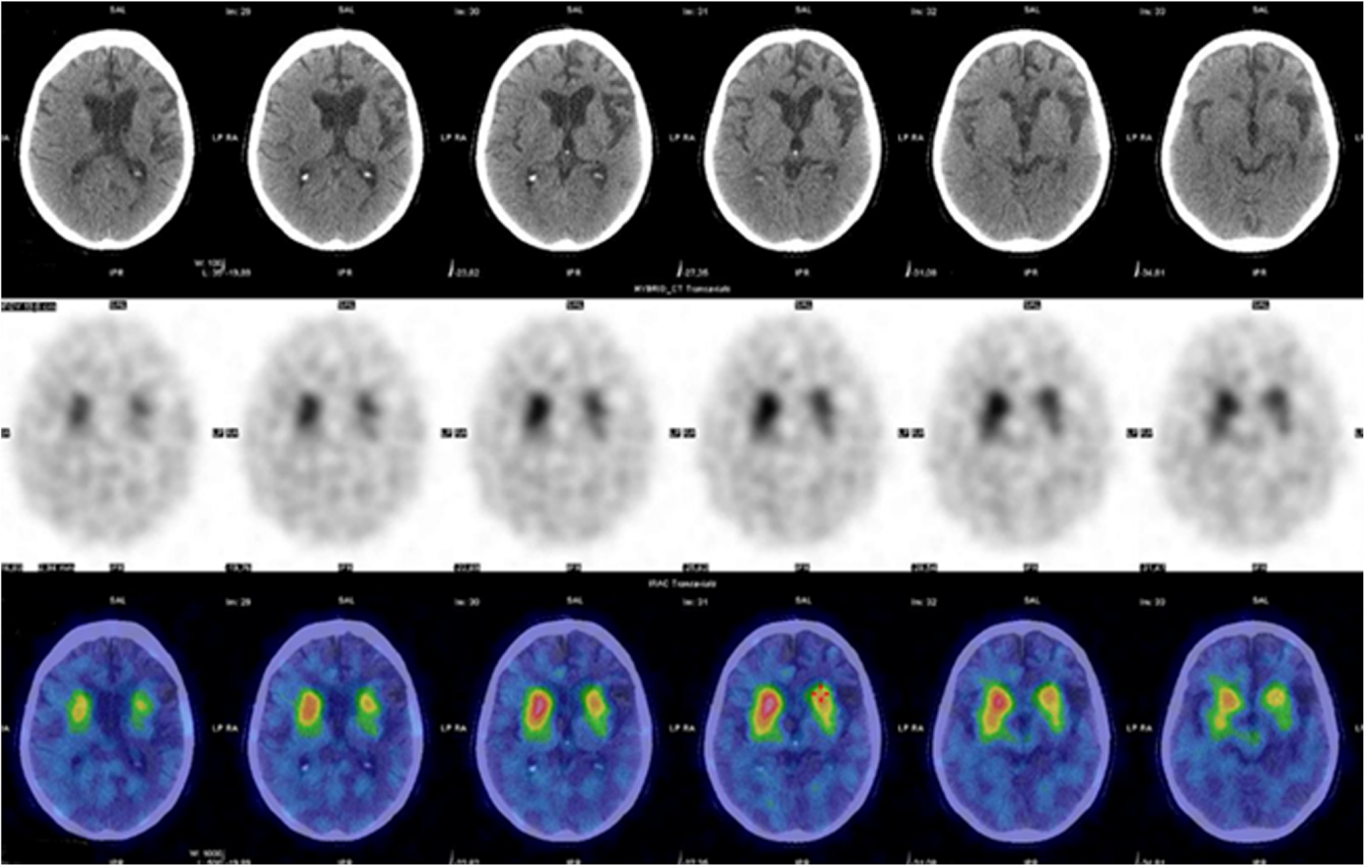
^123^I-FP-CIT SPECT/CT in a patient with parkinsonism. A 63-year-old man with parkinsonian symptoms for the past 12 months, initially diagnosed as Parkinson’s disease but with no appreciable response to L-DOPA treatment, was referred to brain scintigraphy. Transaxial SPECT and SPECT/CT (centre and bottom row) show reduced tracer uptake in the left putamen. CT (top row) demonstrates ipsilateral cortical atrophy. These combined findings suggest a diagnosis of corticobasal atrophy with associated parkinsonism, rather than typical Parkinson’s disease. *[Images provided courtesy of Dr. Duccio Volterrani, Regional Center of Nuclear Medicine, University of Pisa, Italy]*
